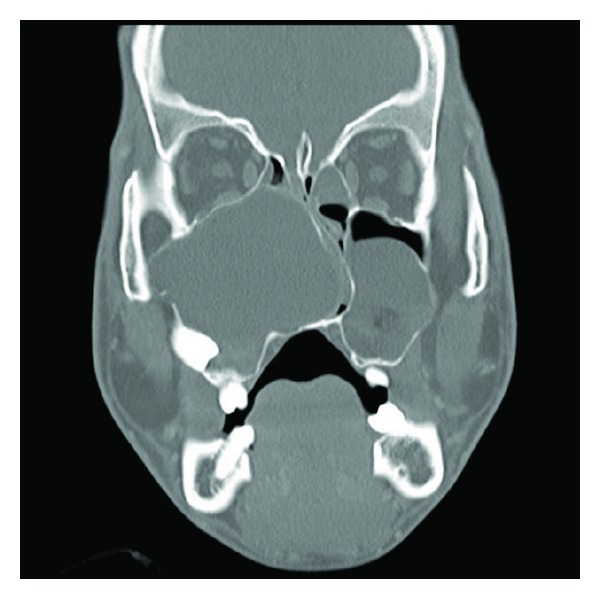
A preoperative coronal computed tomography scan shows a large cystic lesion involving the right maxillary sinus and inferior nasal meatus.
